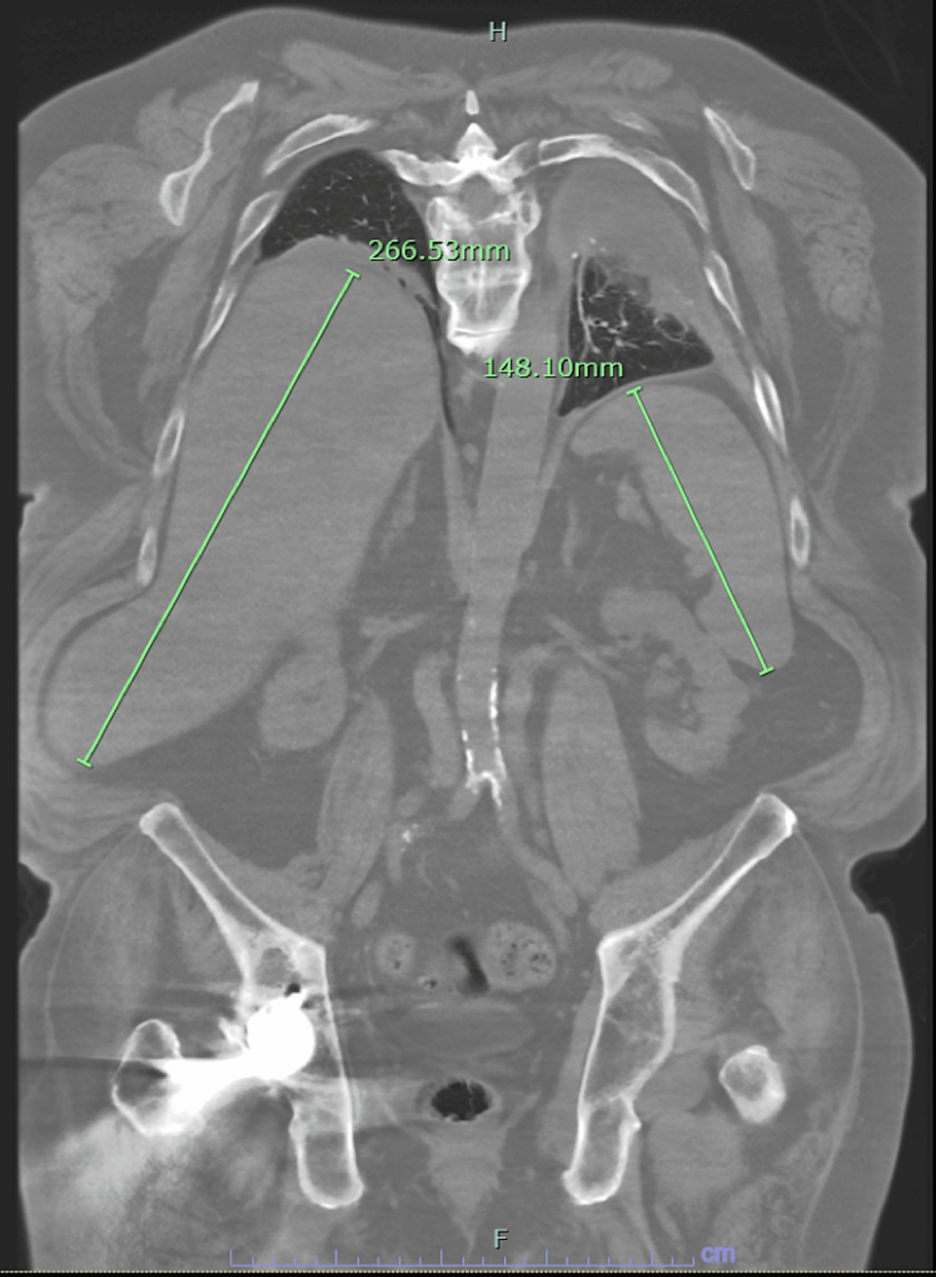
CT imaging showing hepatosplenomegaly CT: computed tomography
